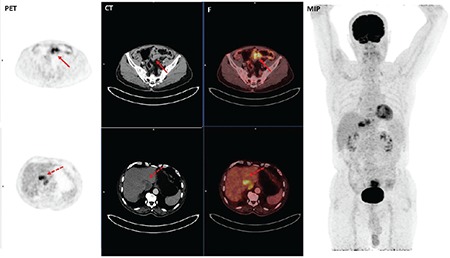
A 70-year-old male patient with wild type adenocarcinoma localized to sigmoid colon positron emission tomography, computed tomography, Fusion, maximum intensity projection. Red arrows show fluorodeoxyglucose uptake of the primary tumor. Primary tumor: SUV_max_: 11.5 dotted red arrows show hepatic metastases SUV_max_: Maximum standardized uptake value, PET: Positron emission tomography, CT: Computed tomography, F: Fusion, MIP: Maximum intensity projection
